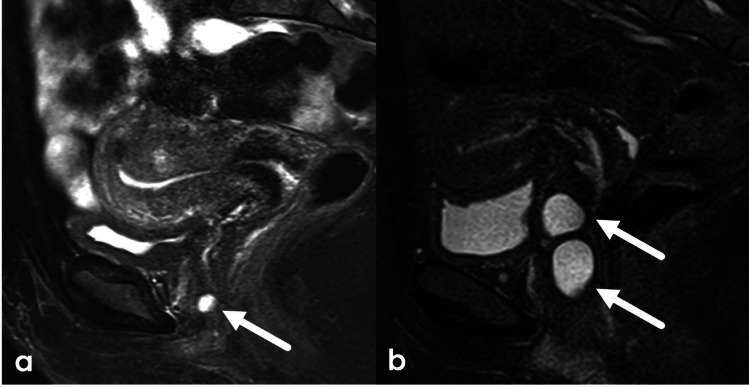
Sagittal T2WI with fat saturation demonstrating Gartner duct cysts in two separate patients: 37-year-old undergoing MRI of the pelvis for abnormal uterine bleeding (a), Gartner duct cyst incidentally noted, and (b) 29-year-old female with vaginal mass noted on annual pelvic exam
